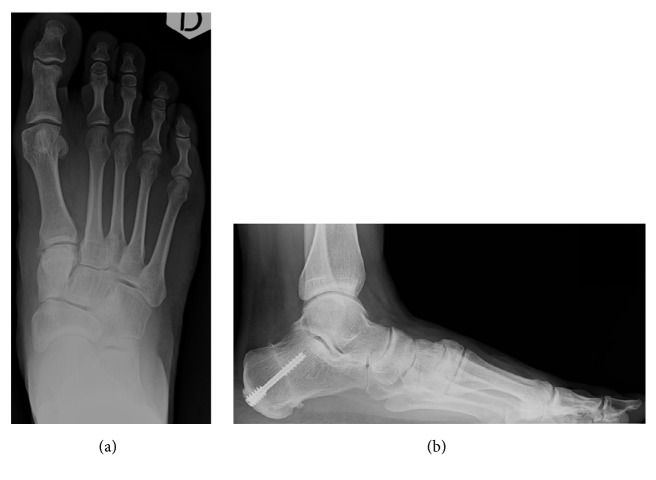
Postoperative (bone allograft) anterior-posterior (a) and lateral (b) weight-bearing radiographs.
